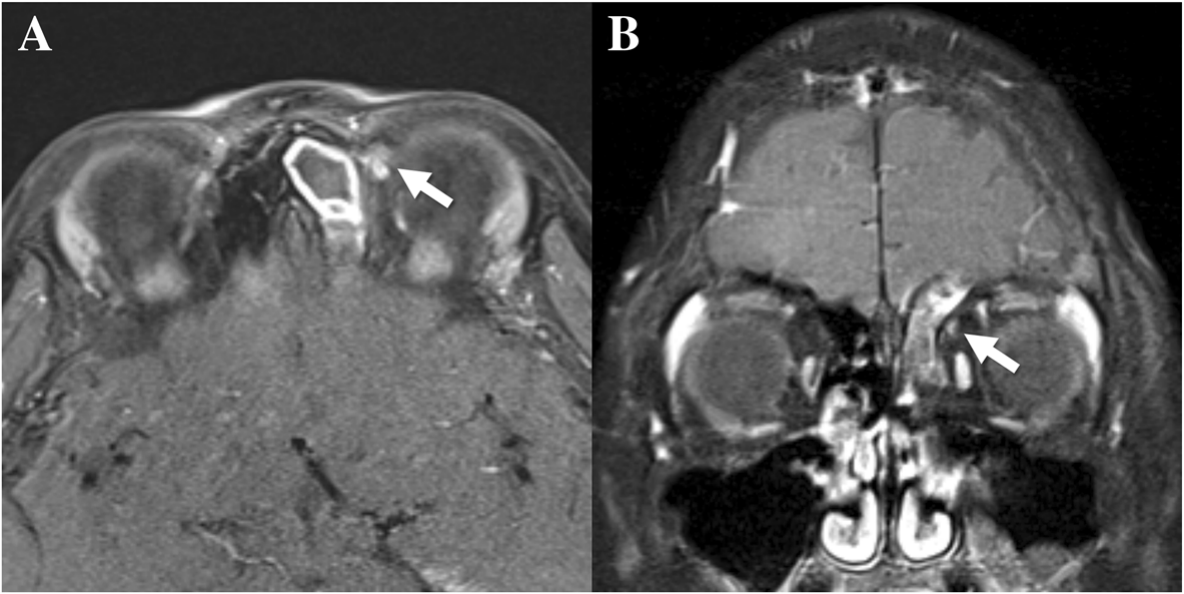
Gadolinium-enhanced T1 MRI demonstrating enhancement on the left trochlea (arrows). Frontal, ethmoidal, and maxillary sinusitis is also observed. a axial image, b coronal image
